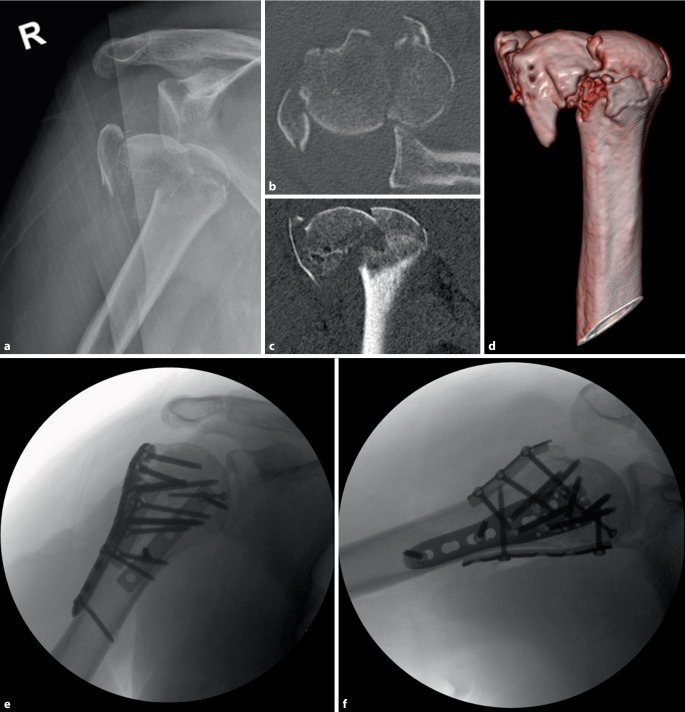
Aufnahmen eines 55-jährigen Patienten mit anteriorer Luxationsfraktur Typ 5 nach Resch et al. [22] nach einem Treppensturz. a Präoperative Röntgenbildgebung, b axiale Schicht der CT-Bildgebung, c koronare Schicht der CT-Bildgebung, d Volumenrekonstruktion der proximalen Humerus, e intraoperative a.-p.-Röntgenaufnahme, f intraoperative axiale Röntgenaufnahme
